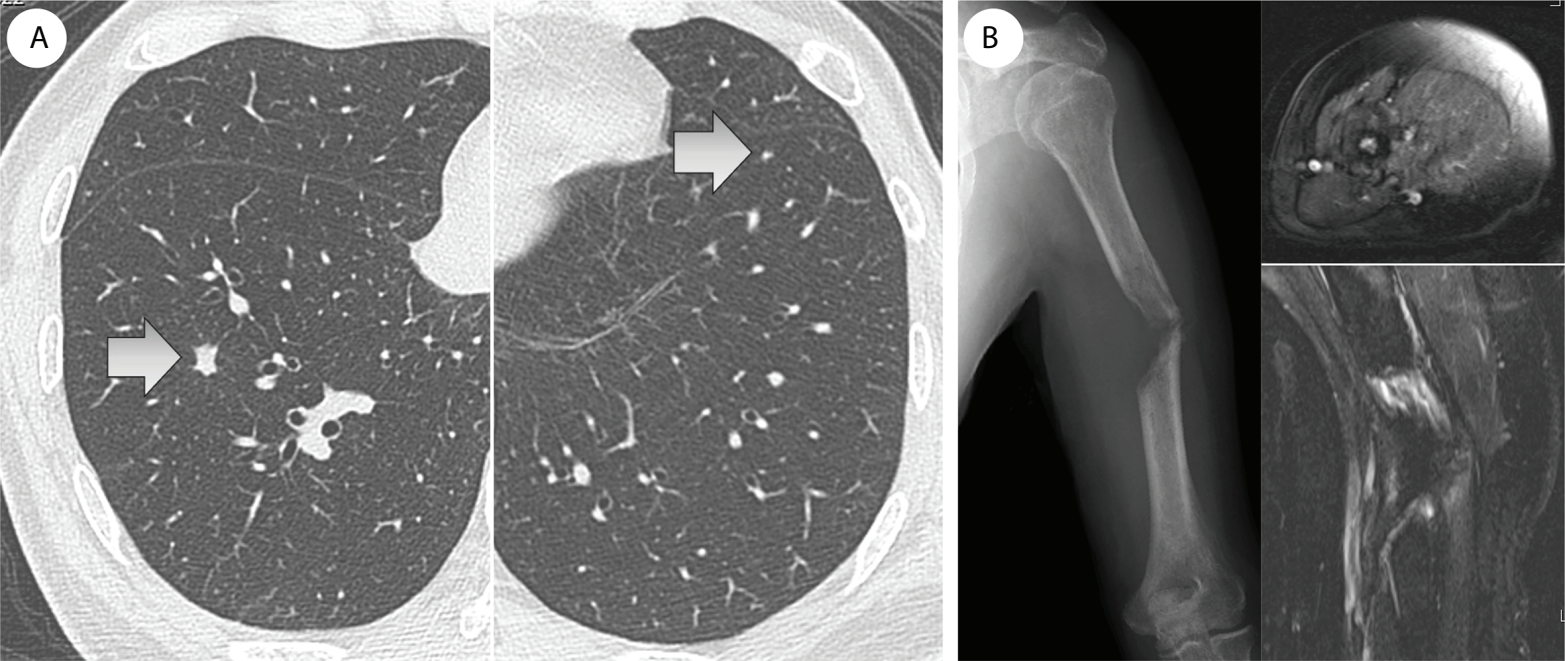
(A) After four courses of ICI treatment, the pulmonary metastases had almost disappeared. (B) Furthermore, the tumor at the fracture site had shrunk remarkably and almost disappeared (MRI: gadolinium-enhancement).
